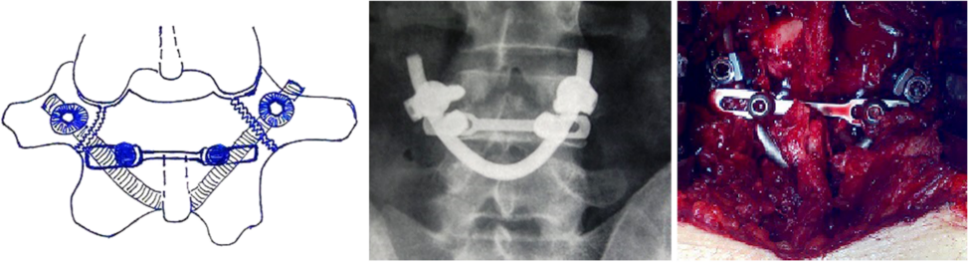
Lumbar midline sagittal T2-weighted magnetic resonance imaging (center) of Case one showing no degenerated disc, frank instability, or multilevel disease; and extension standing lateral and oblique X-rays (left, right) showing the pars defect with no segmental instability.
